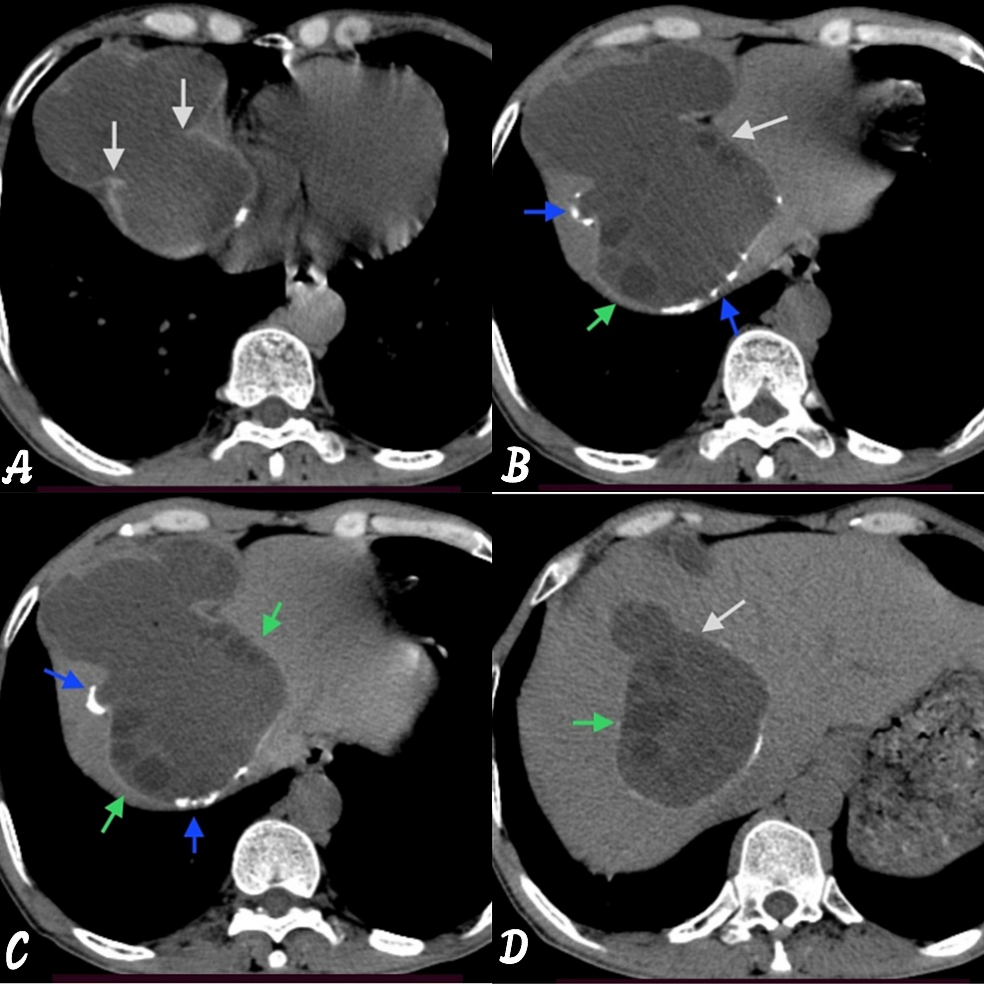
Axial CT images showing hydatid cyst reaching up to right atrium. Axial images showing (A) incomplete membrane within the hydatid cyst (white arrow); (B and C) incomplete calcifications (blue arrow) and daughter cyst (green arrow); and (D) incomplete membrane within the hydatid cyst (white arrow) and daughter cyst (green arrow).
